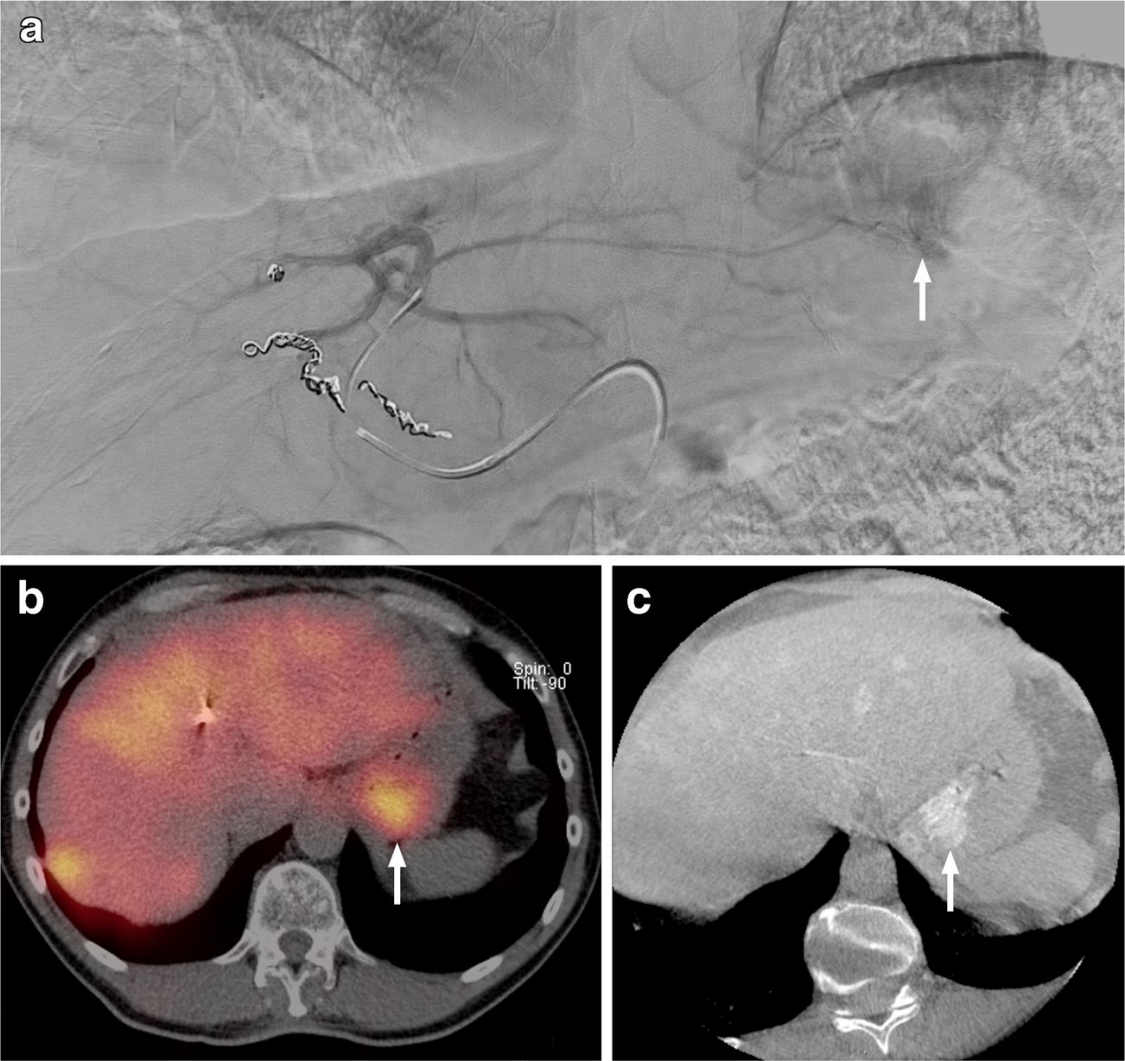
A 56-year-old male with a primary rectal neuroendocrine tumour, liver and bone metastases. (a) Digital subtraction angiography of ^166^Ho scout dose procedure. (b) Corresponding ^166^Ho scout dose SPECT/CT. (c) Corresponding cone beam CT. All images show extrahepatic deposition and contrast blush in gastric fundus (arrows). After coiling of accessory left gastric artery, extrahepatic deposition on SPECT/CT and contrast blush on cone beam CT disappeared (images not shown). Accessory left gastric artery originated distally from left hepatic artery, running through the ligamentum venosum towards the gastric fundus [16]
